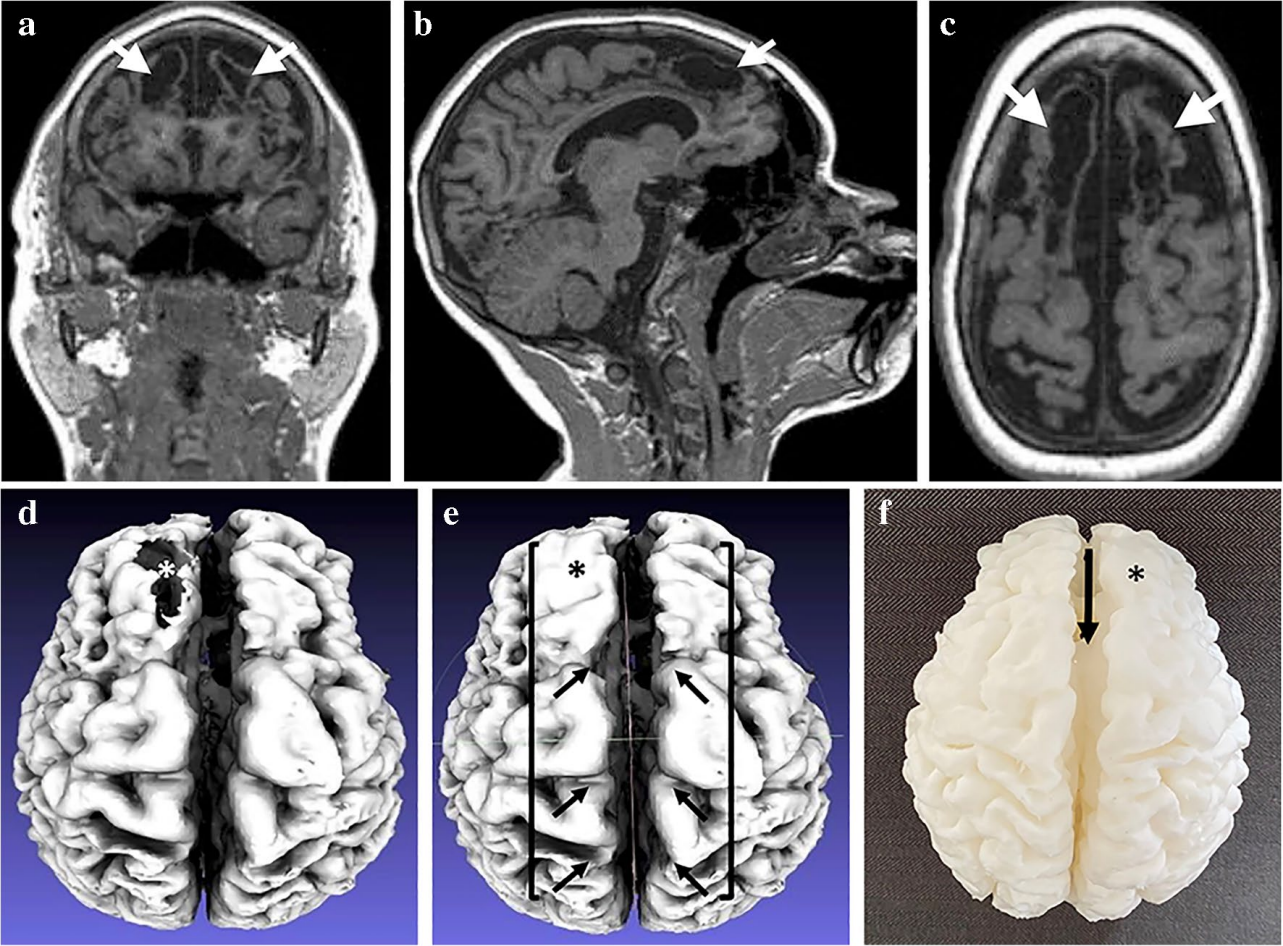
A 10-year-and-5-month-old girl with a previous global partial-prolonged hypoxic ischemic injury affecting the parasagittal watershed regions. a–c Multiplanar coronal (a), sagittal (b) and axial (c) reconstructions of T1 weighted magnetic resonance images demonstrate bilateral parasagittal cystic encephalomalacia (*arrows*) and very thin residual cortex of the superior frontal lobes. d Vertex view of an initial standard tessellation language (STL) surface model which was unsuccessful in demonstrating the thin cortical mantle of the right frontal lobe, leaving a large hole with a view into the cystic cavity involving the right frontal lobe white matter (*asterisk*). e Vertex view of a subsequent usable STL surface model created by patching the hole using manual editing (*asterisk*). Significant parasagittal watershed (between *brackets*) atrophy is accurately demonstrated (*arrows*). f Vertex view of the final 3-D print model shows the interhemispheric widening (*arrow*) and the area that was patched within the right frontal lobe (*asterisk*)
